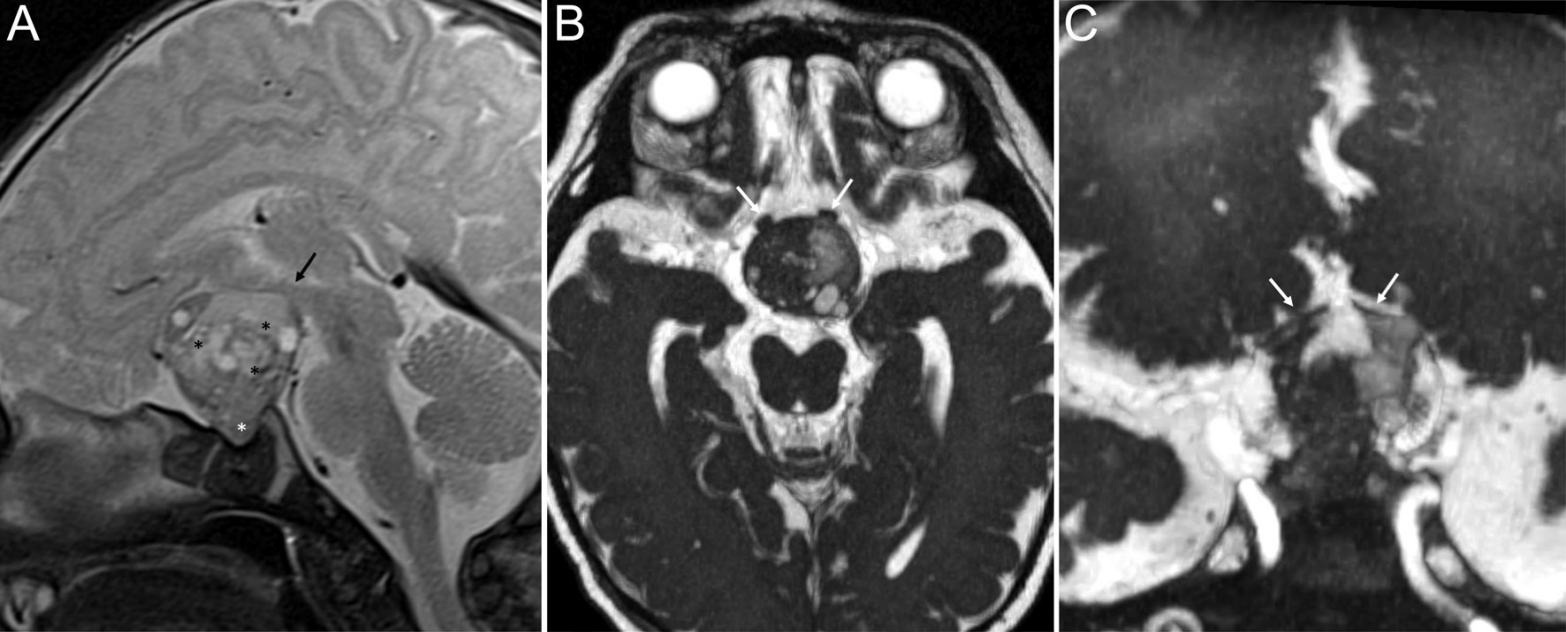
Magnetic resonance imaging (MRI) of a patient with perinatally diagnosed congenital craniopharyngioma (case 1). MRI at the fourth day of life demonstrating a cCP of intermediate size having more solid than cystic parts. The main part is in suprasellar location (A: black asterisk), only a small part is reaching intrasellarly (A: white asterisk). The optic chiasm and optic nerves are elevated and compressed (B and C: white arrows) and the posterior part is compressing the region of the mammillary bodies (A: black arrow) indicating an involvement of the posterior hypothalamus (A: sagittal T2 WI, sagittal and high resolution 3D-T2 WI with B: axial and C: coronal reconstruction).
